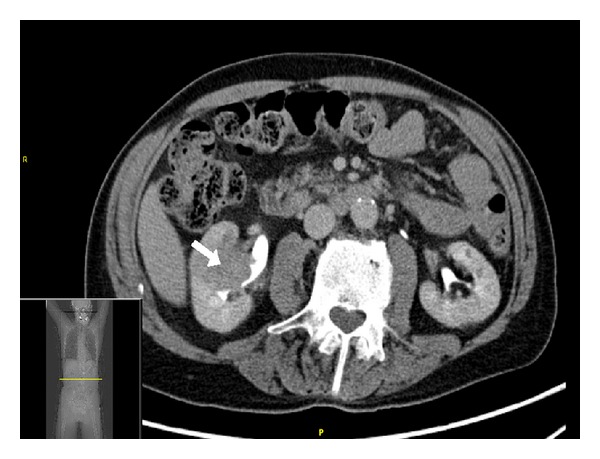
Computed tomography scan showing cross-section of the abdomen with a right-sided renal mass measuring 3.8 cm.
